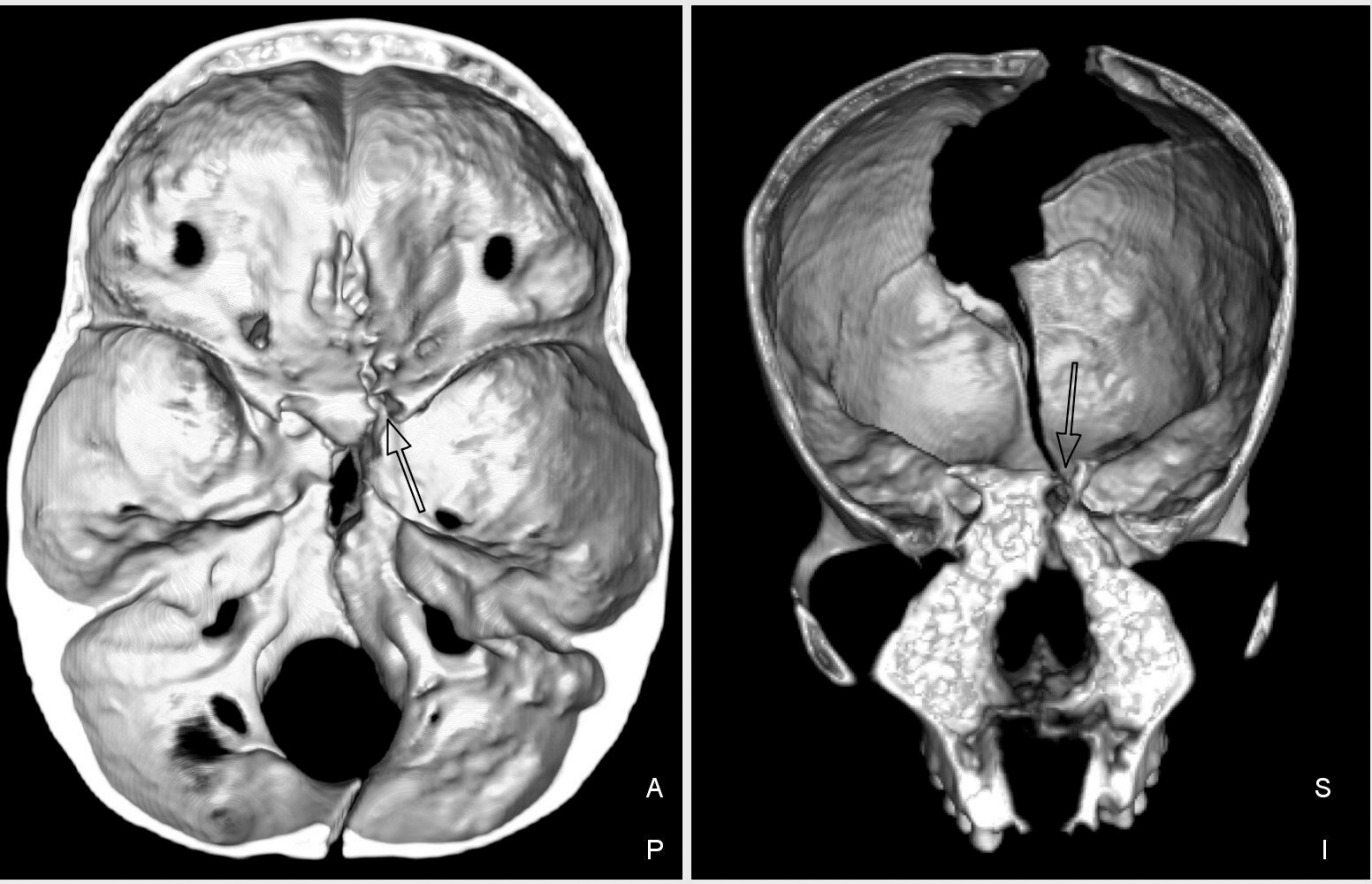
CT scan of Individual 1, SAM-AP 6421, showing cleaved sphenoid.
